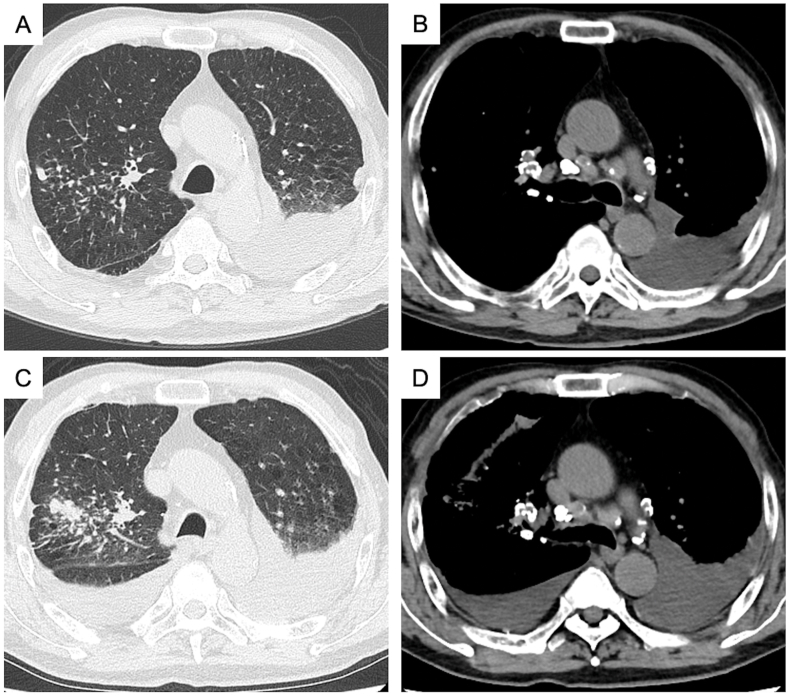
(A–B) and (C–D) are chest computed tomography (CT) images acquired at the first visit and 2 years and 4 months after the first visit, respectively. CT images acquired at the first visit show small nodules in the right upper lung fields (A) and left-sided pleural effusion and egg-shell calcification of the hilar and mediastinal lymph nodes (B). CT images acquired at the second visit show an increase in the sizes of the small nodules (C) and the amount of pleural effusion bilaterally (D).
